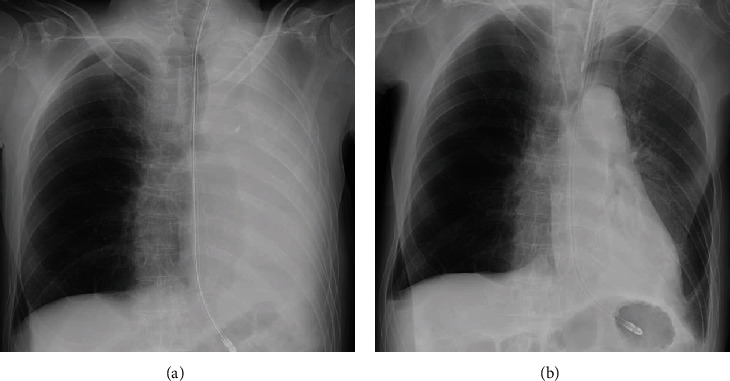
Chest radiographs obtained (a) before and (b) after treatment with sputum aspiration and retrieval of the valproic acid tablet using bronchoscopy.
